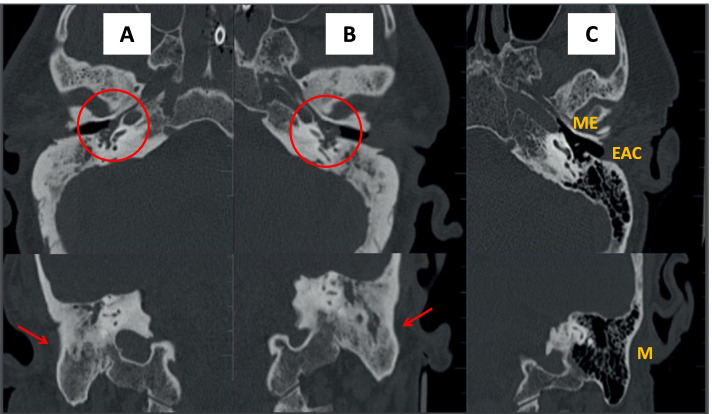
Computed tomography (CT) image of pathological petrous bone [A = right; B = left] compared to a normal CT (C). Axial images (superior) and coronal images (inferior). Bilateral invasion of the middle ear (red circle), mastoid cavity, and mastoid air cells (red arrow)
